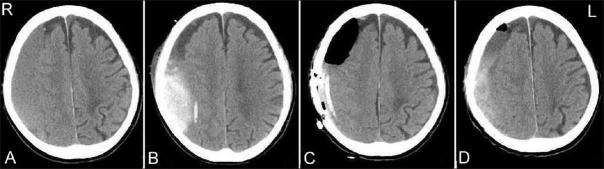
CT images of case 2. (A) CT scan on admission showing right CSH. (B) CT scan 1 day after the surgery, showing a bleeding between the dura mater and the outer membrane. (C) CT scan after the craniotomy. (D) CT scan 2 weeks after the craniotomy, showing an increase in right subdural hematoma
